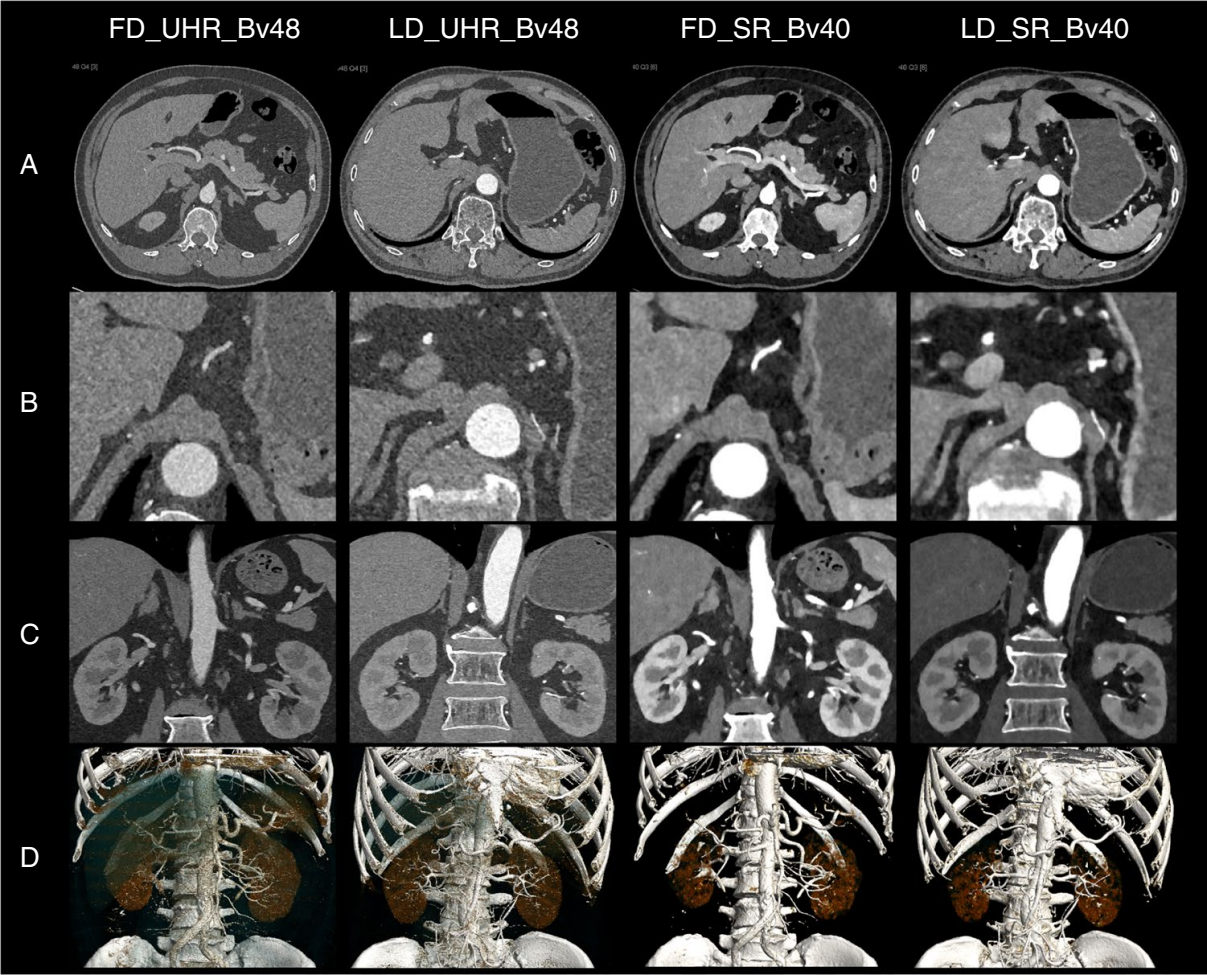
Representative example of two participants who underwent full-dose and low-dose scan. A 61-year man with a BMI of 29.8 kg/m^2^ underwent abdominal CTA using a FD protocol with CTDIvol, DLP, SSDE, and effective dose of 8.75 mGy, 245.0 mGy·cm, 10.50 mGy, and 3.68 mSv, respectively (the first and the third column). Another 62-year man with a BMI of 23.2 kg/m^2^ underwent abdominal CTA using a LD protocol with CTDIvol, DLP, SSDE, and effective dose of 3.60 mGy, 106.0 mGy·cm, 5.05 mGy, and 1.59 mSv, respectively (the second and the fourth column). The data were reconstructed into FD_UHR_Bv48, LD_UHR_Bv48, FD_SR_Bv40, LD_SR_Bv40 images, respectively. A The overall quality of FD and LD images were comparable. B–D The artery visibility of FD and LD images were comparable regardless of axial, coronal and volume-rendered images. The UHR images allowed more vascular segments and thinner image slice but with higher image noise. The SR images showed better contrast but lower vessel sharpness and thicker image slice
